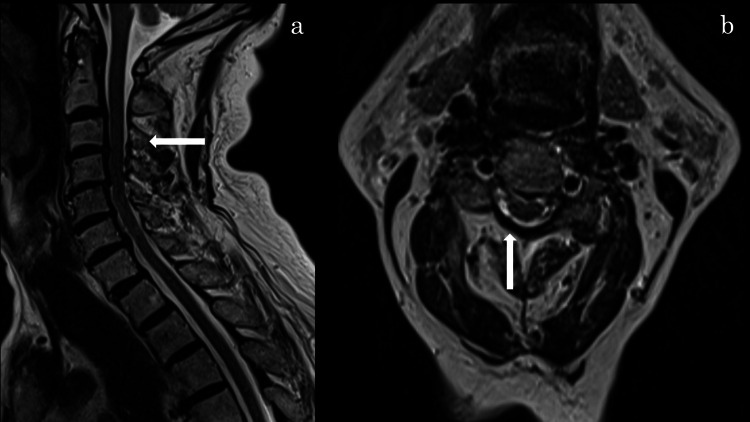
MRI T2-weighted images Sagittal (a) and axial (b) T2-weighted images showing changes following edoxaban discontinuation at the C2–C4 levels.
